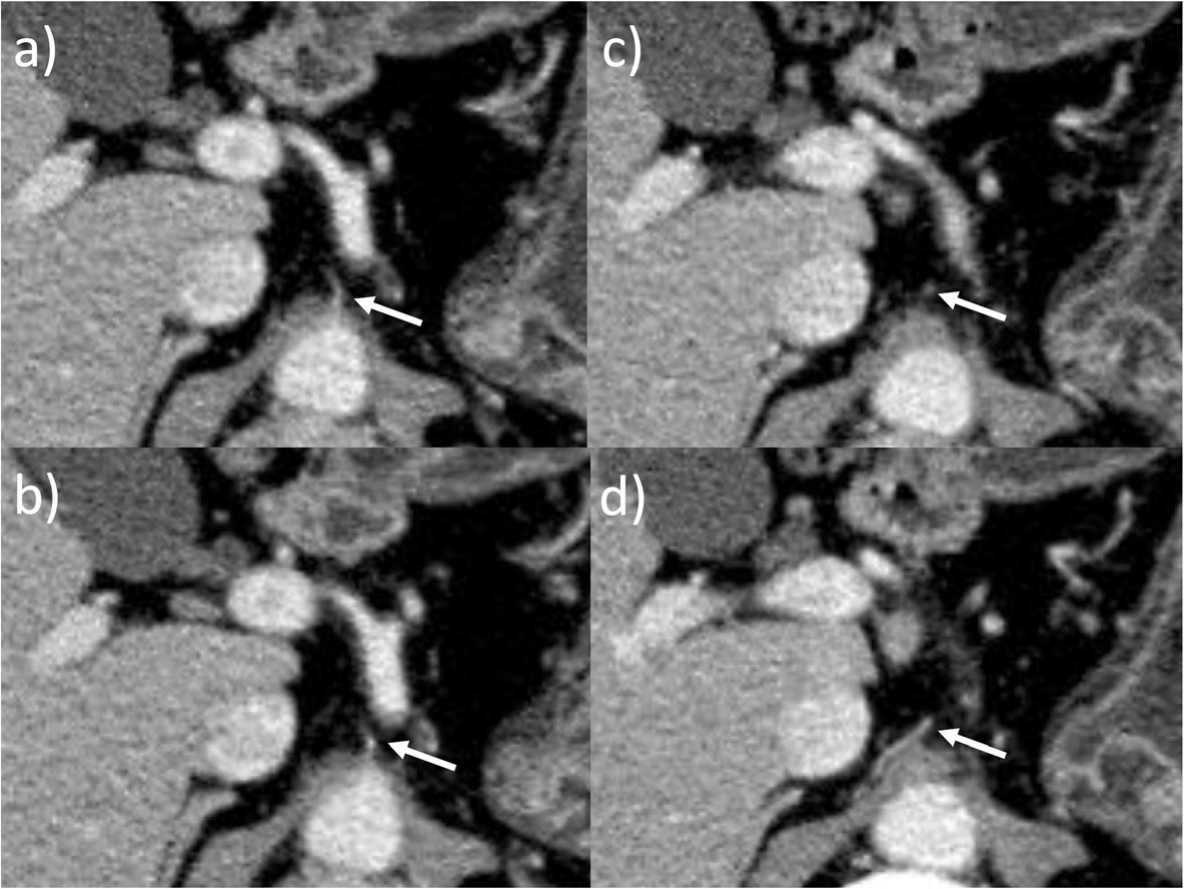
Preoperative CT. a The RIPA (arrow) originated from the aorta without a common trunk. b, c The RIPA ran close to the celiac artery. d The RIPA ran across and in front of the crus
